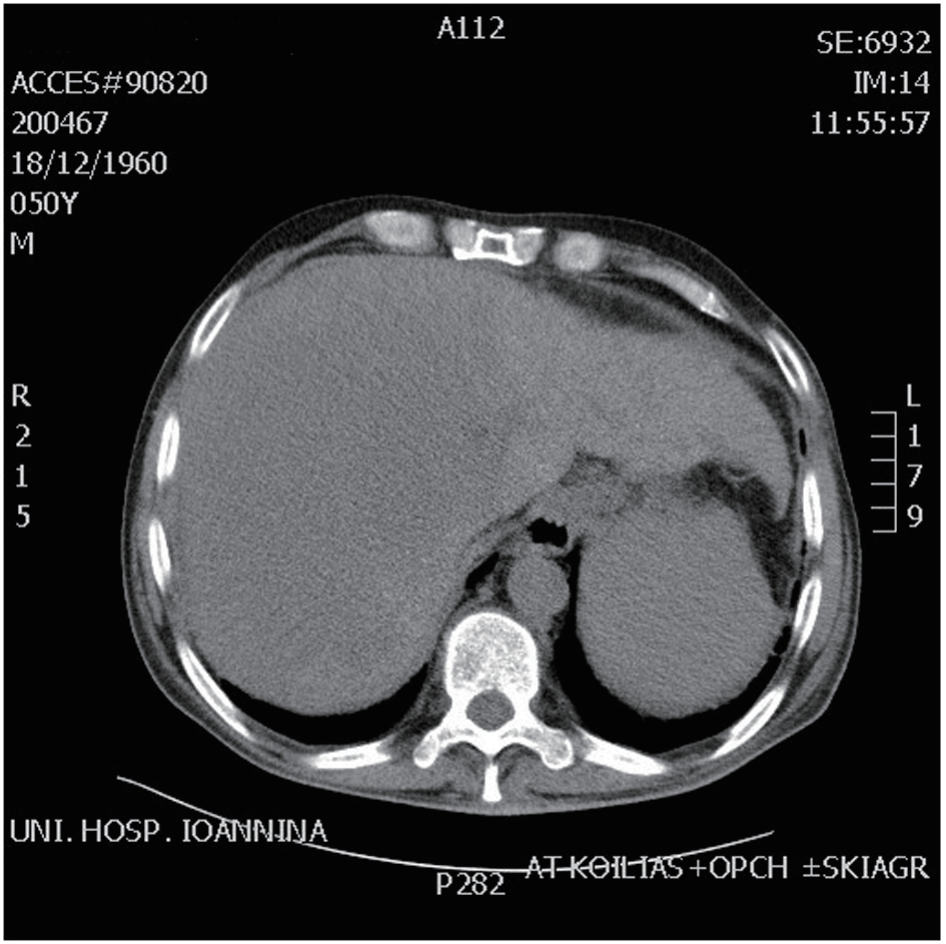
CT scan abdomen showing huge mass in the right lobe of liver.
